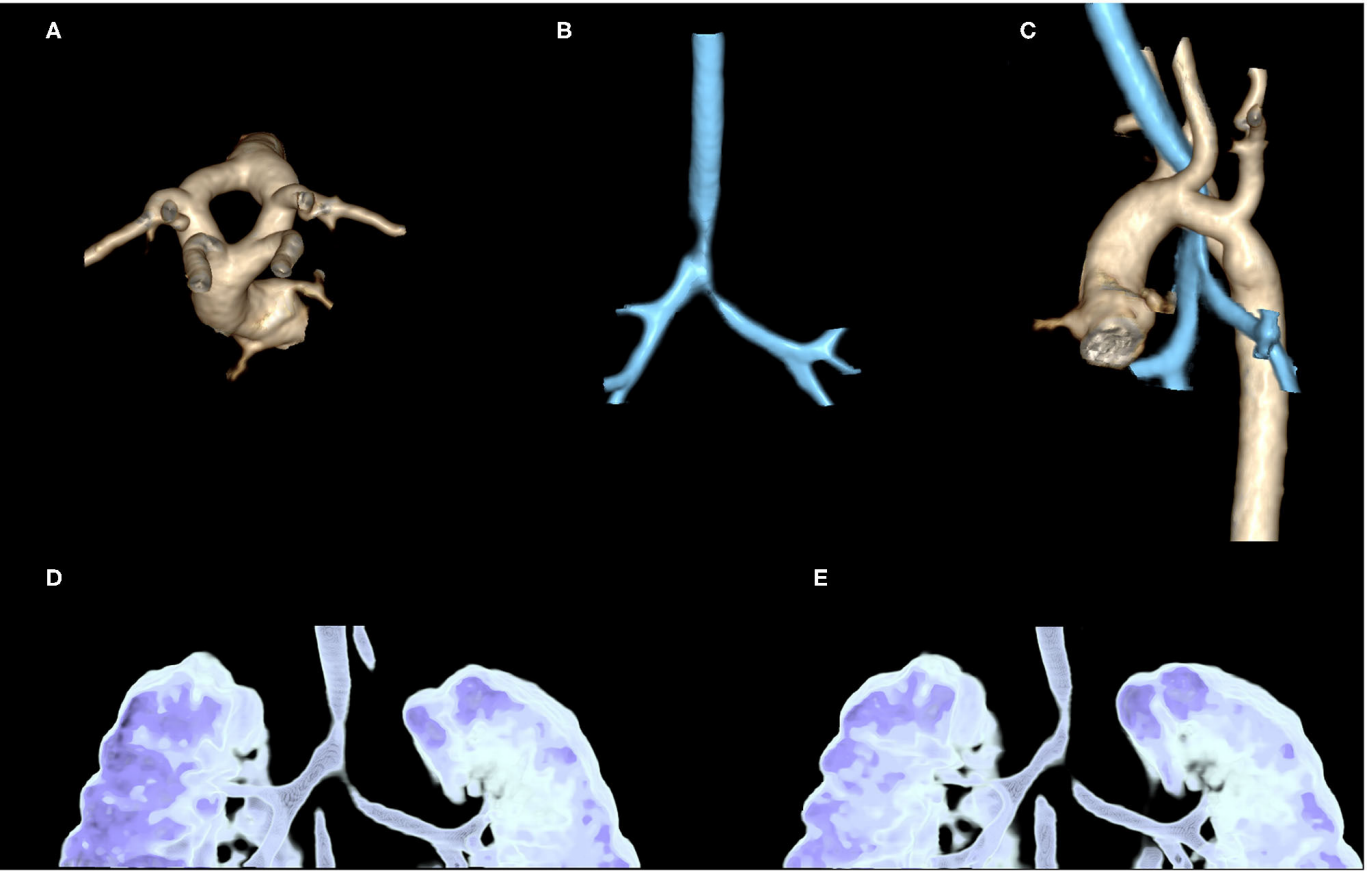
Contrast-enhanced CT in a 2-month-old girl, referred to our hospital after prenatal diagnosis of vascular ring: three-dimensional (3D) volume rendering of thoracic aorta confirms the presence of a double aortic arc with two arches (left and right) equally sized (A). 3D volume rendering of airways shows stenosis of distal trachea until carina, near the vascular ring (B). An evaluation of these stenoses compared to thoracic aorta shows that they are, respectively, at the level of the vascular ring and left arterial duct (C). 3D volume rendering of the dynamic expiratory phase displays associated signs of tracheobronchomalacia of distal trachea and left-sided bronchus with significant variation of caliber between the inspiratory (D) and expiratory phase (E).
